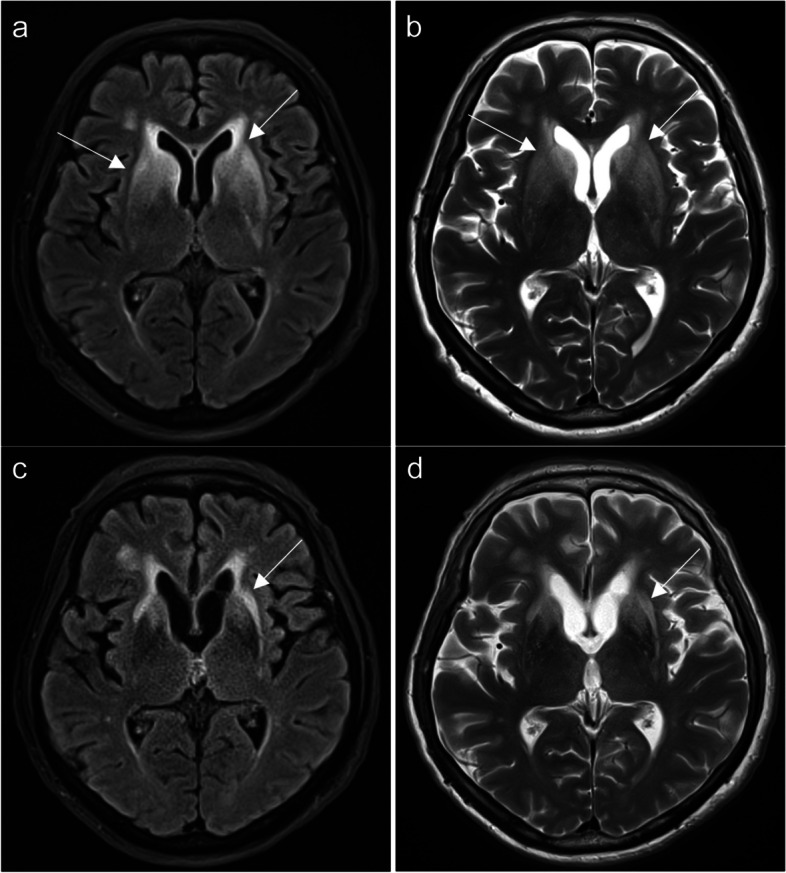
Pembrolizumab-induced toxic leukoencephalopathy. A 72-year-old female with non-small cell lung carcinoma (NSCLC) had received Pembrolizumab (200 mg intravenous administered every 3 weeks). Three days after the second cycle of Pembrolizumab, she was admitted with sudden onset confusion, ataxia, and cognitive impairment. Axial FLAIR (a) and T2-weighted (b) MRI brain scans reveal hyperintensities in bilateral periventricular white matter and bilateral lentiform nuclei, external capsules, and claustrum (arrows). These findings are consistent with Pembrolizumab-induced toxic leukoencephalopathy. She was given high-dose methylprednisolone administered intravenously at 1000 mg daily for 3 days, with a near-complete resolution of his expressive dysphasia and improvement in gait. She was discharged on a regimen of 60 mg of prednisolone daily, which was subsequently weaned by 10 mg each week. A Follow-up MRI brain one month after discharge reveals a significant reduction of the T2 and FLAIR hyperintensities in the basal ganglia as demonstrated on axial FLAIR (c) and T2-weighted (d) images
